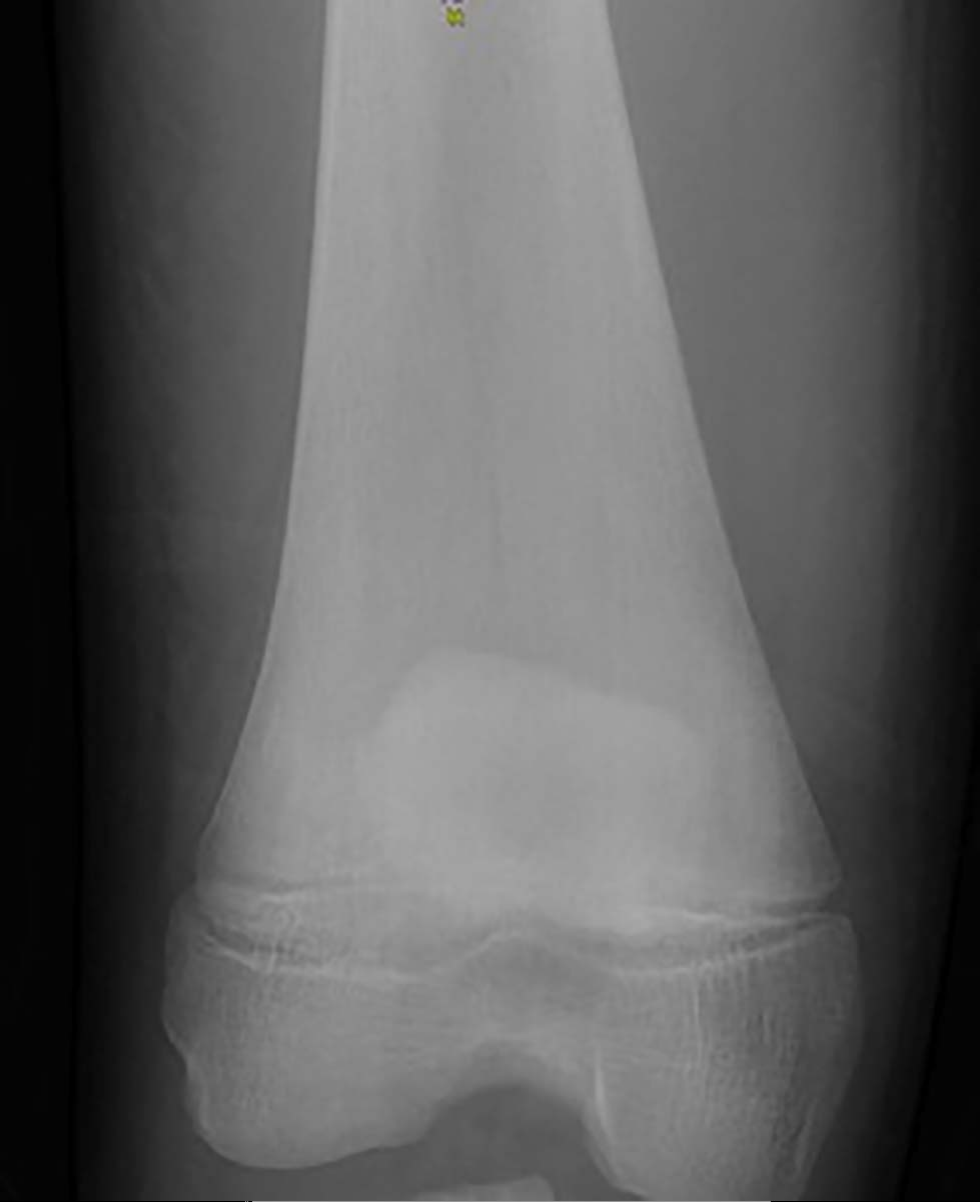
Radiograph showing the Erlenmeyer flask deformity of the distal femur.
